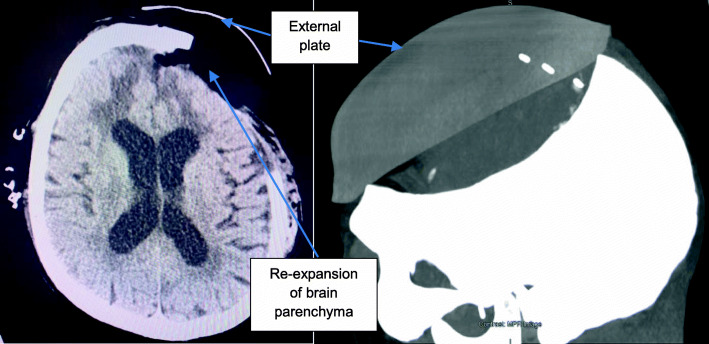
CT head scan with 3D reconstruction two days after device application. As the brain surface expands towards the inner surface of the cranioplasty, the ventricles become enlarged. This is an *ex vacuo* phenomenon and does not represent hydrocephalus
